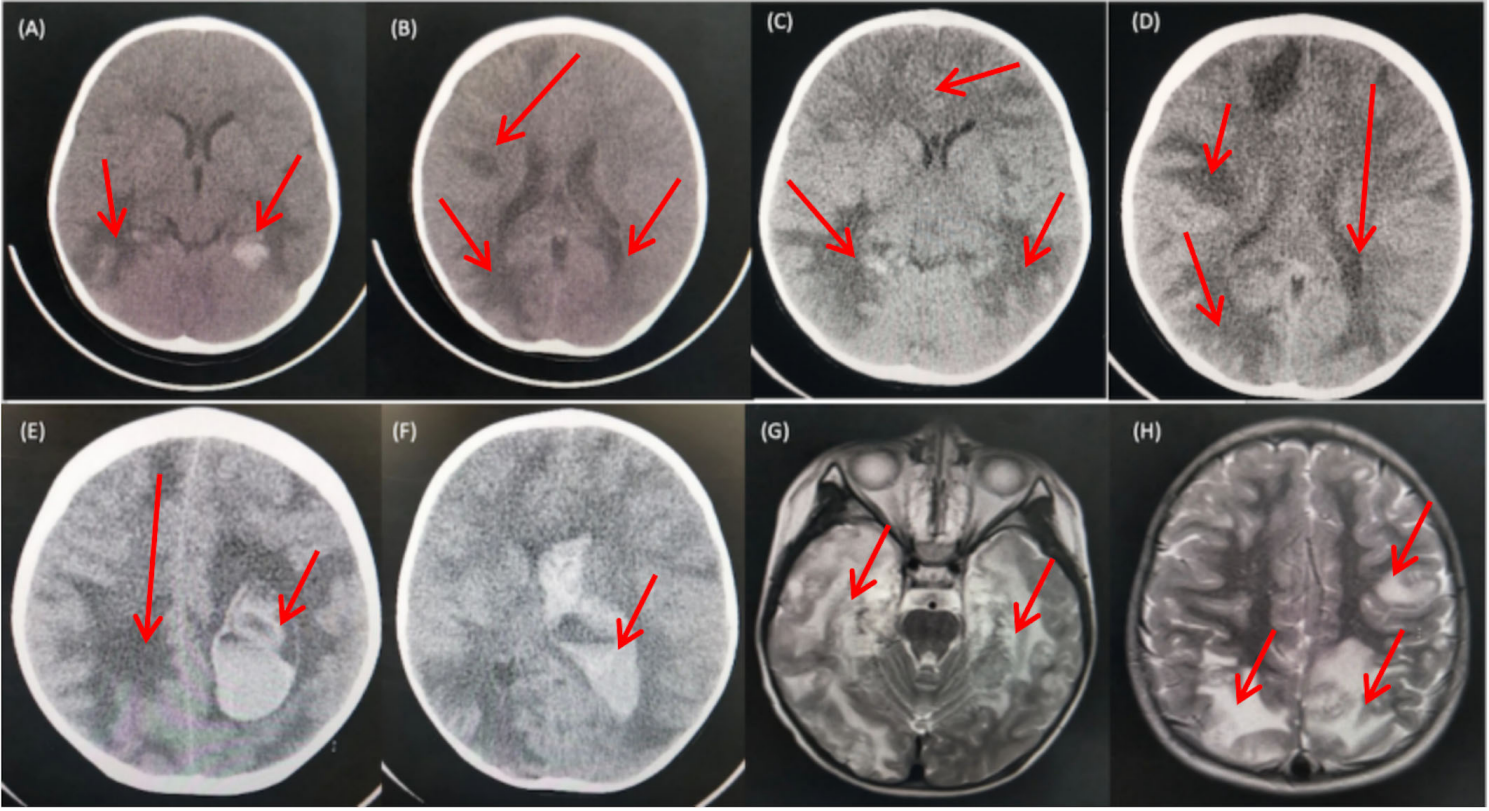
CT and MRI Findings of the Patient. (A, B) CT scans obtained prior to admission revealed multiple low-density patchy shadows in the bilateral frontal, parietal, temporal, and occipital lobes, with high-density patchy shadows observed in the bilateral temporal and occipital lobes. (C, D) CT scans obtained on day 16 of admission showed a decrease in density in the right occipital lobe and progressive involvement in the remaining lobes. (E, F) CT scans obtained on day 18 of admission revealed diffuse brain swelling, ventricular hemorrhage, subdural hemorrhage, and increased hemorrhage in the left parietal lobe. (G, H) MRI findings from the second day of admission demonstrated lesions with high intensities on T2-weighted images in the bilateral deep temporal and parietal lobes.
